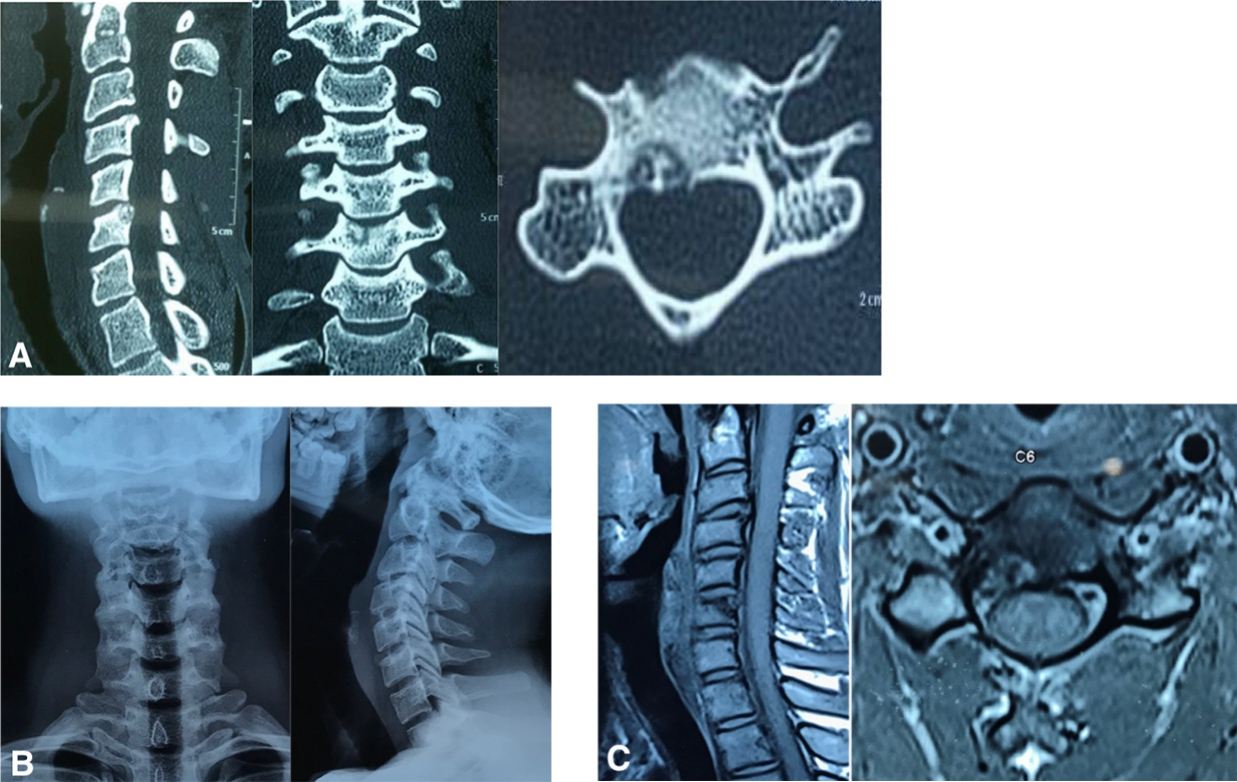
(a) Preoperative CT scan with the nidus in C6 vertebral body abutting the end plate and posterior wall. (b) Plain X-rays not depicting the lesion. (c) MRI T1 sagittal and axial images showing the lesion with peripheral edema.
